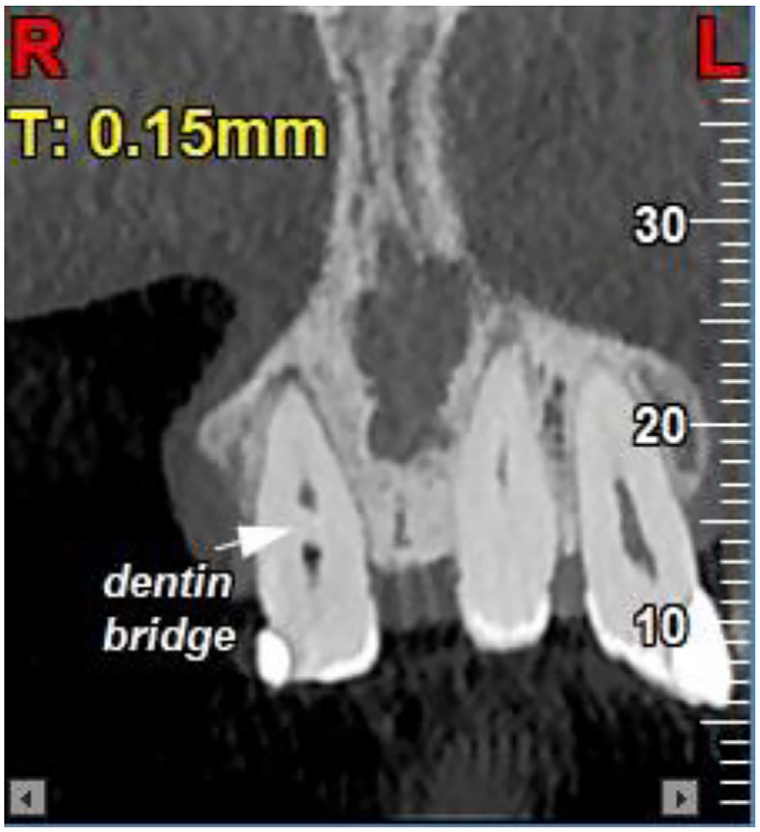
Coronal view of CBCT image shows dentin bridge separating the necrotic coronal pulp from the vital apical pulp.
